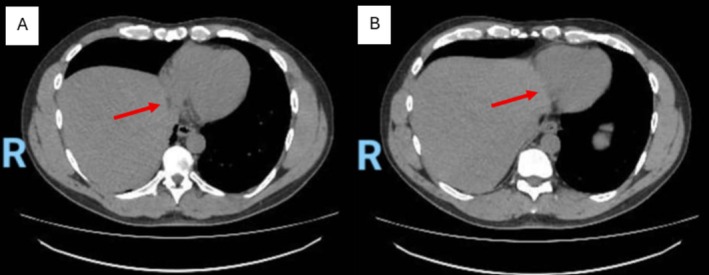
(Placeholder image for Video 1): Axial view of the thoracic CT scan, showing the liver compressing the right atrium (arrow).
